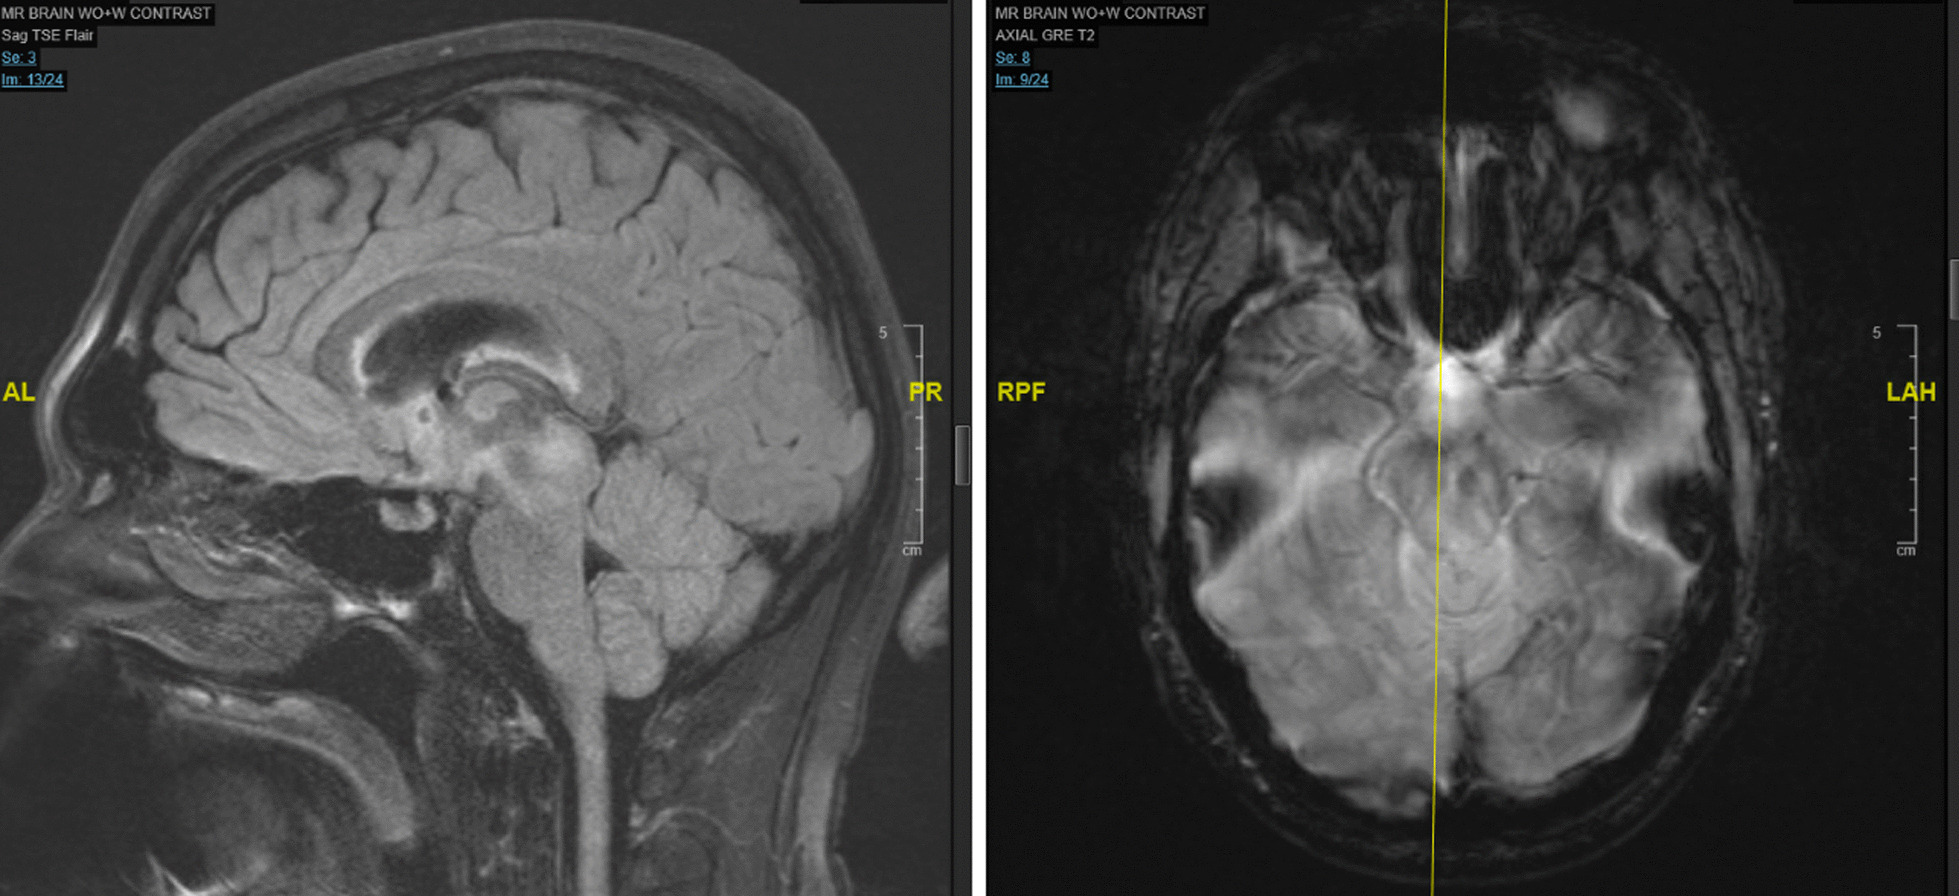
Initial brain magnetic resonance imaging showing T2 prolongation involving the bilateral mesial temporal lobes, hippocampi/fornices, hypothalamus, mammillary bodies and mammillothalamic tracts, and midbrain including periaqueductal gray
